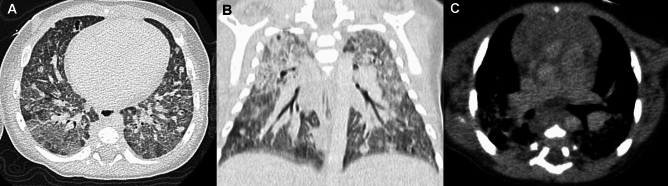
Langerhans cell histiocytosis (LCH). A 2-year-old boy with fever and lymphadenopathy. A,B CT depicted reticulo-nodular shadows predominantly in the upper and middle lung fields, intermingled with small cavitary lesions. C CT also showed thymic enlargement with heterogeneous internal densities and spotty calcifications. The thymic calcifications indicate a high likelihood of LCH
